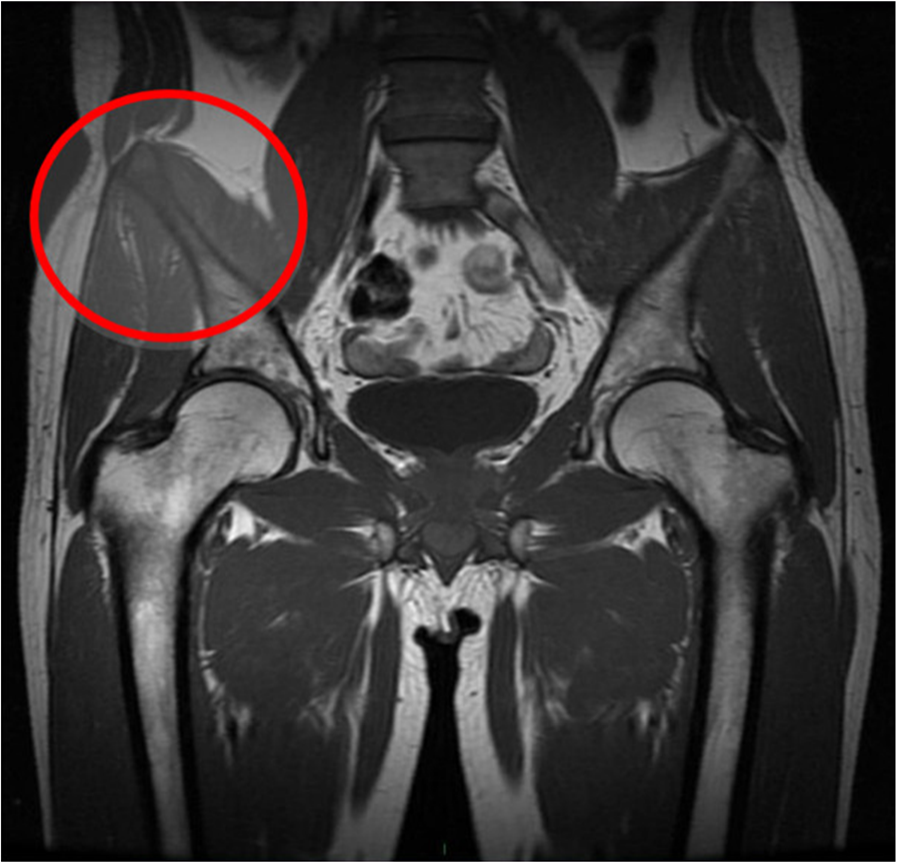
Coronal MRI showing the involvement of the right iliac crest.
